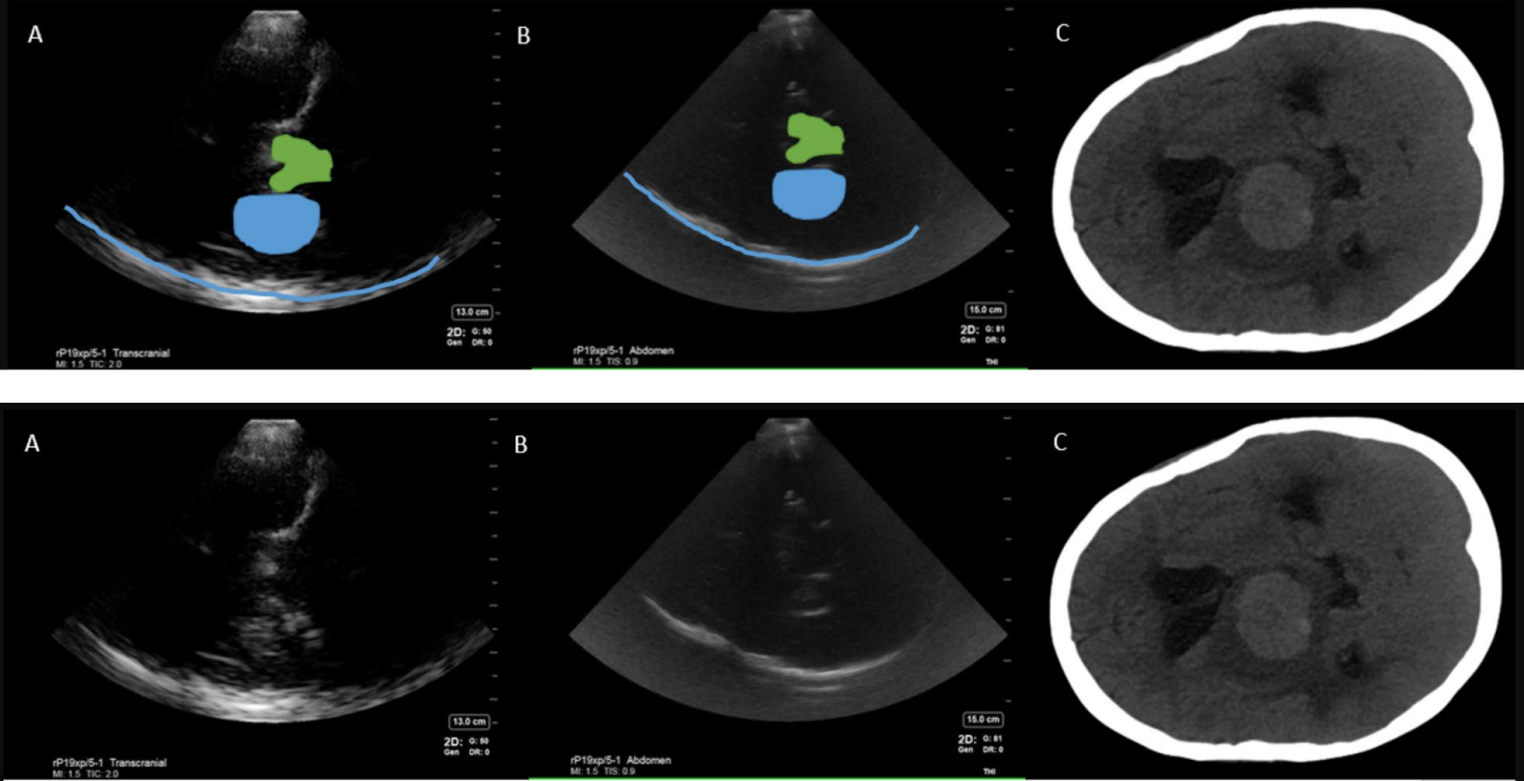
Thalamic tumor creating a hyperechoic signal similar to intracerebral haemorrhage. A—Transcranial preset, B—abdominal preset, C—computed tomography (CT) brain scan. Blue line—opposite skull, green—midbrain. Blue shape outlines the hyperechoic signal corresponding to the tumor on CT scan
